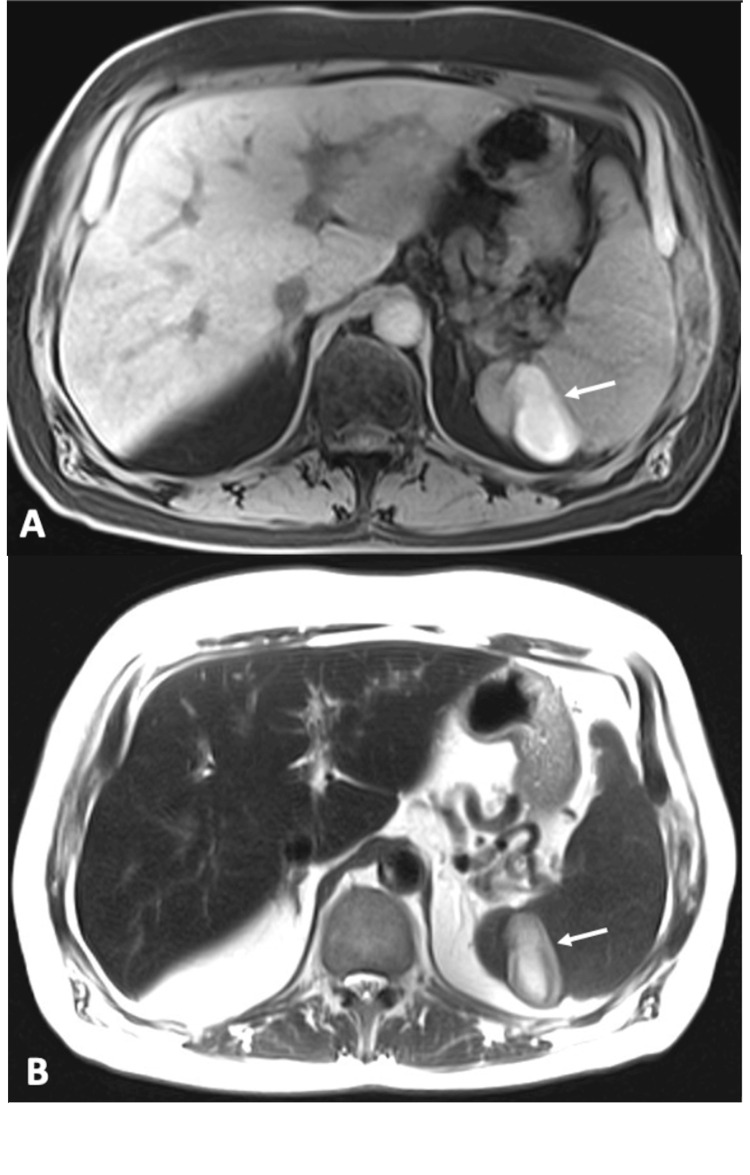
Axial T1-weighted fat-saturated (A) and axial T2-weighted (B) MR images demonstrated a 4.8 x 2.6 cm infarct in the spleen (arrows).
